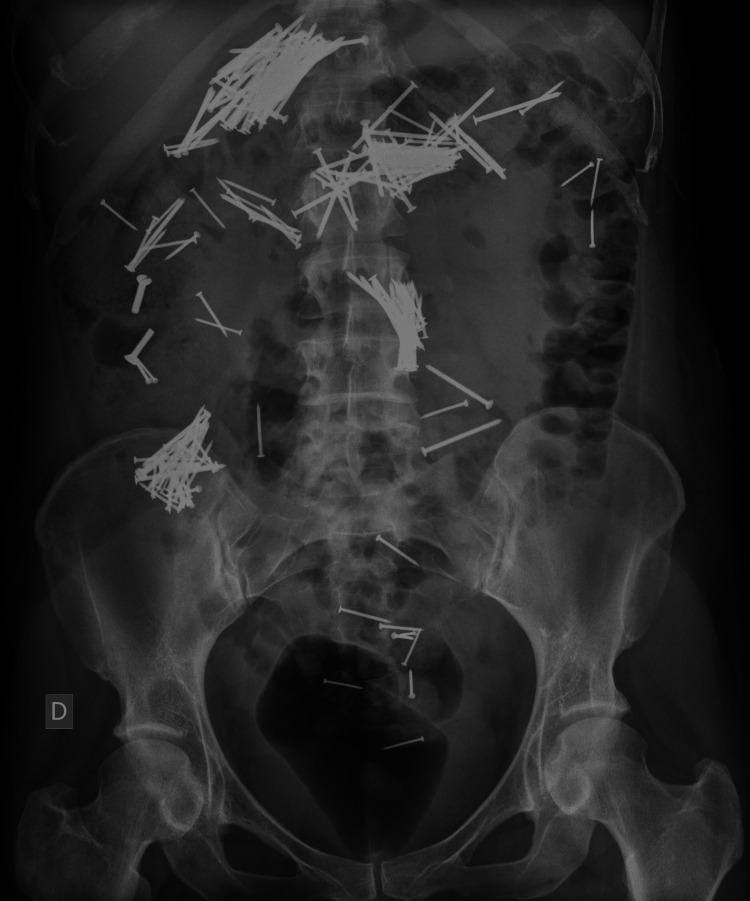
Abdominal X-ray upon arrival. Supine abdominal radiograph obtained upon presentation shows numerous metallic foreign bodies (nails) dispersed throughout the colon and jejunal loops, indicating widespread gastrointestinal distribution.
